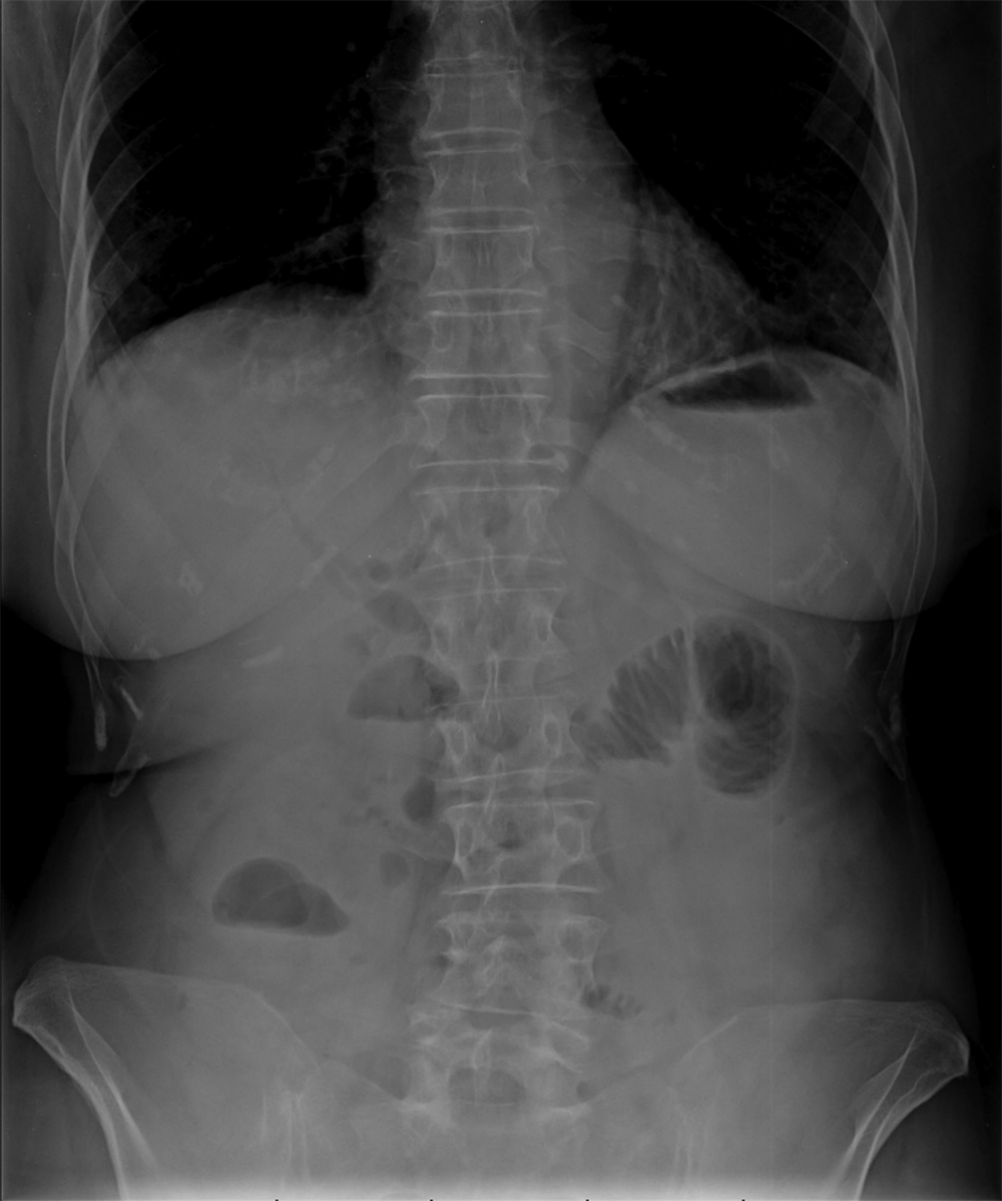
Plain abdominal x-ray showing small bowel dilatation and pneumobilia
